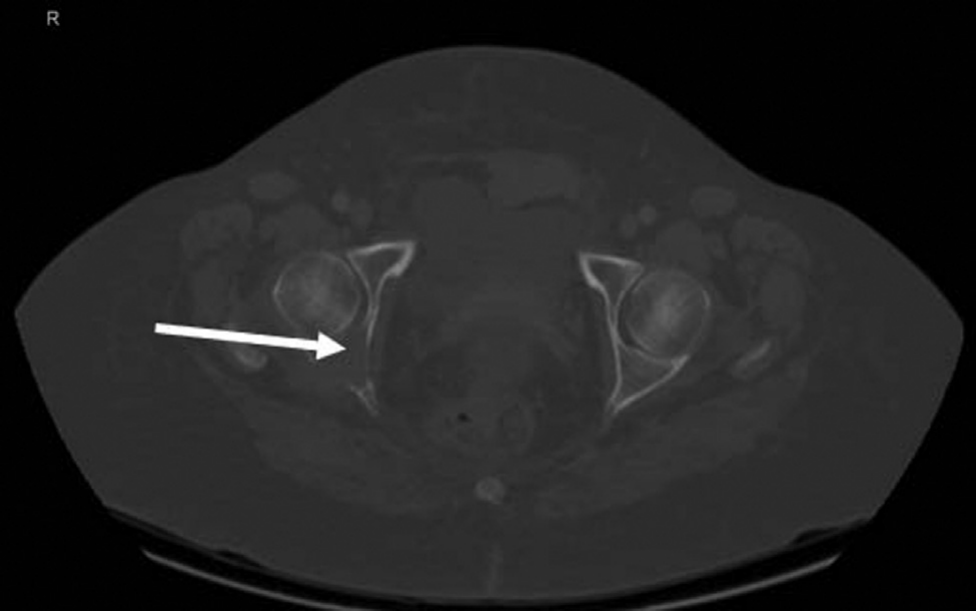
CT scan of the pelvis showing destructive lesion involving the right ischial tuberosity representing A metastatic deposits. CT, computed tomography.
